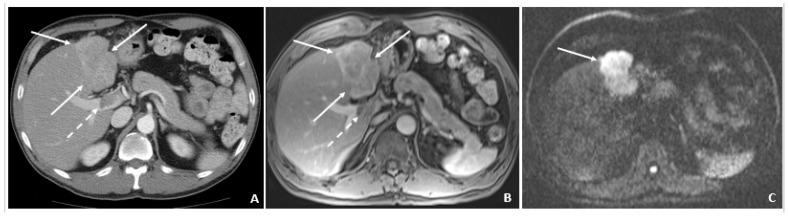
Recurrent HCC 2 Years Later (April 2011). (A) The contrast-enhanced CT image shows a hyperenhancing lesion in the left lobe (arrows) with adjacent tumor thrombus within the portal vein (dashed arrow). (B) The subsequent axial T1-weighted fat-suppressed postcontrast MRI image shows the lesion (arrows) to a slightly better advantage, along with the portal vein tumor thrombus (dashed arrow). (C) The diffusion-weighted image shows marked hyperintensity (arrow) within the left lobar mass, corresponding to diffusion restriction, typical of malignant tumors.
